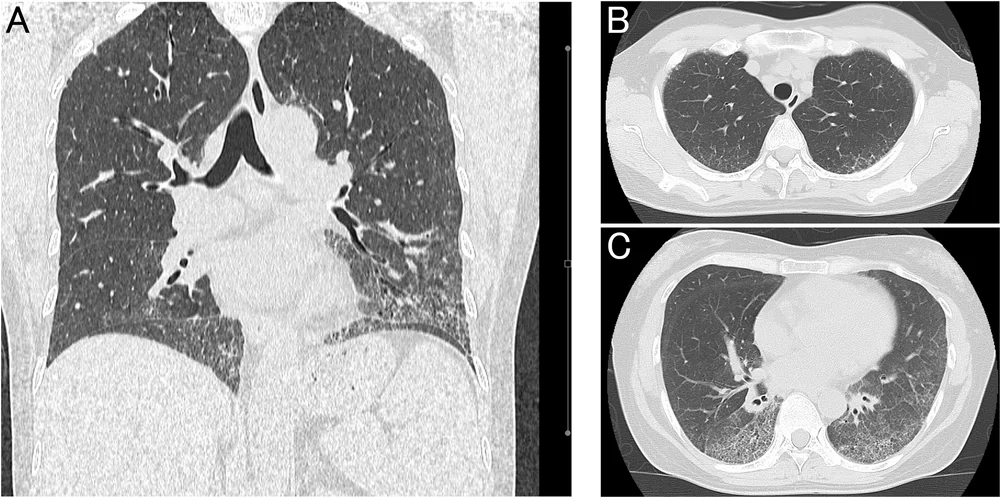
HRCT of the chest. (A) Coronal view shows bilateral peripheral ground‐glass opacities with granular structures, traction bronchiectasis, and reduced lung volume, predominantly in the lower lobes. (B) Axial view of the upper lobe shows subtle subpleural granular opacities. (C) Axial view of the lower lobe demonstrates marked volume loss with reticular opacities and ground‐glass attenuation, consistent with a fibrotic NSIP pattern.
